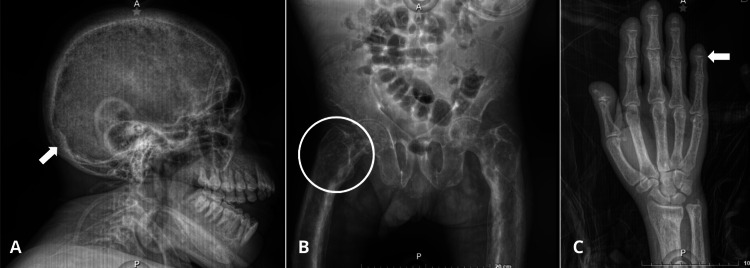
X-rays of the skull, hip, and hand A: Lateral skull X-ray showing calvarial thickening (white arrow) and multiple lytic irregularly circumscribed lesions as well as malocclusion of the upper and lower jaws. B: Anteroposterior hip X-ray showing long bone deformities, areas of osteitis fibrosa cystica (white circle), and O-shaped legs conditioning short stature. C: Anteroposterior X-ray of the hand showing fingertip deformity (white arrow)
